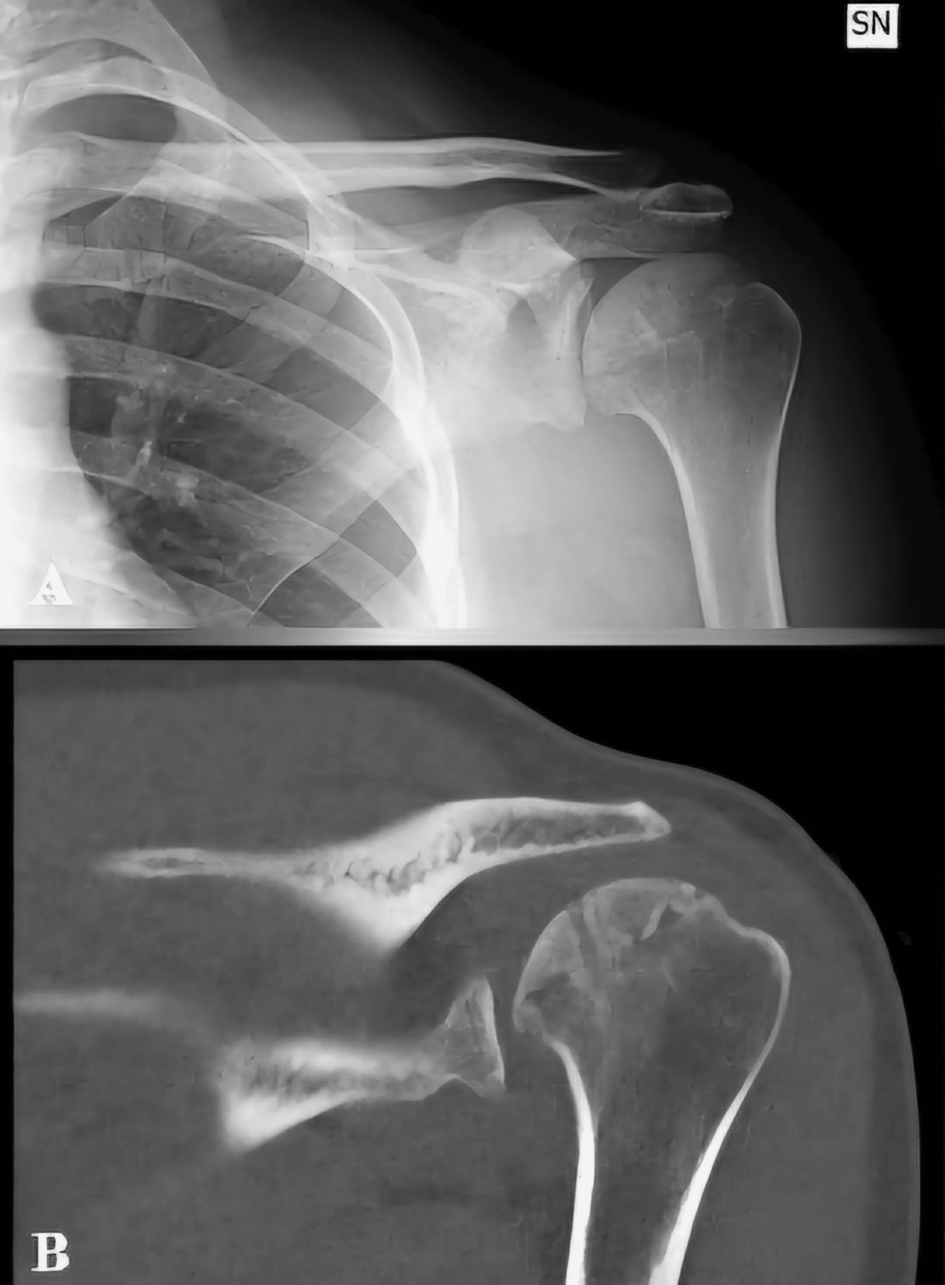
(A) Anteroposterior plain radiograph and (B) CT scan image in coronal plane at sixth-month follow-up.
